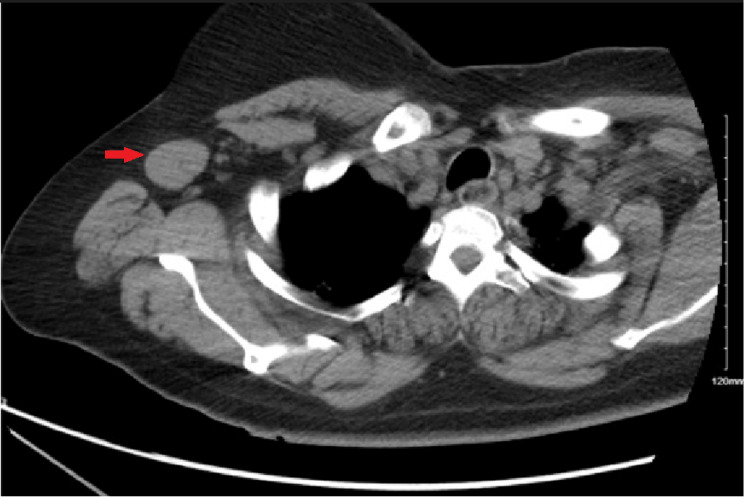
Right axillary lymph node. CT of the chest showing a right axillary lymph node (highlighted by the red arrow).
